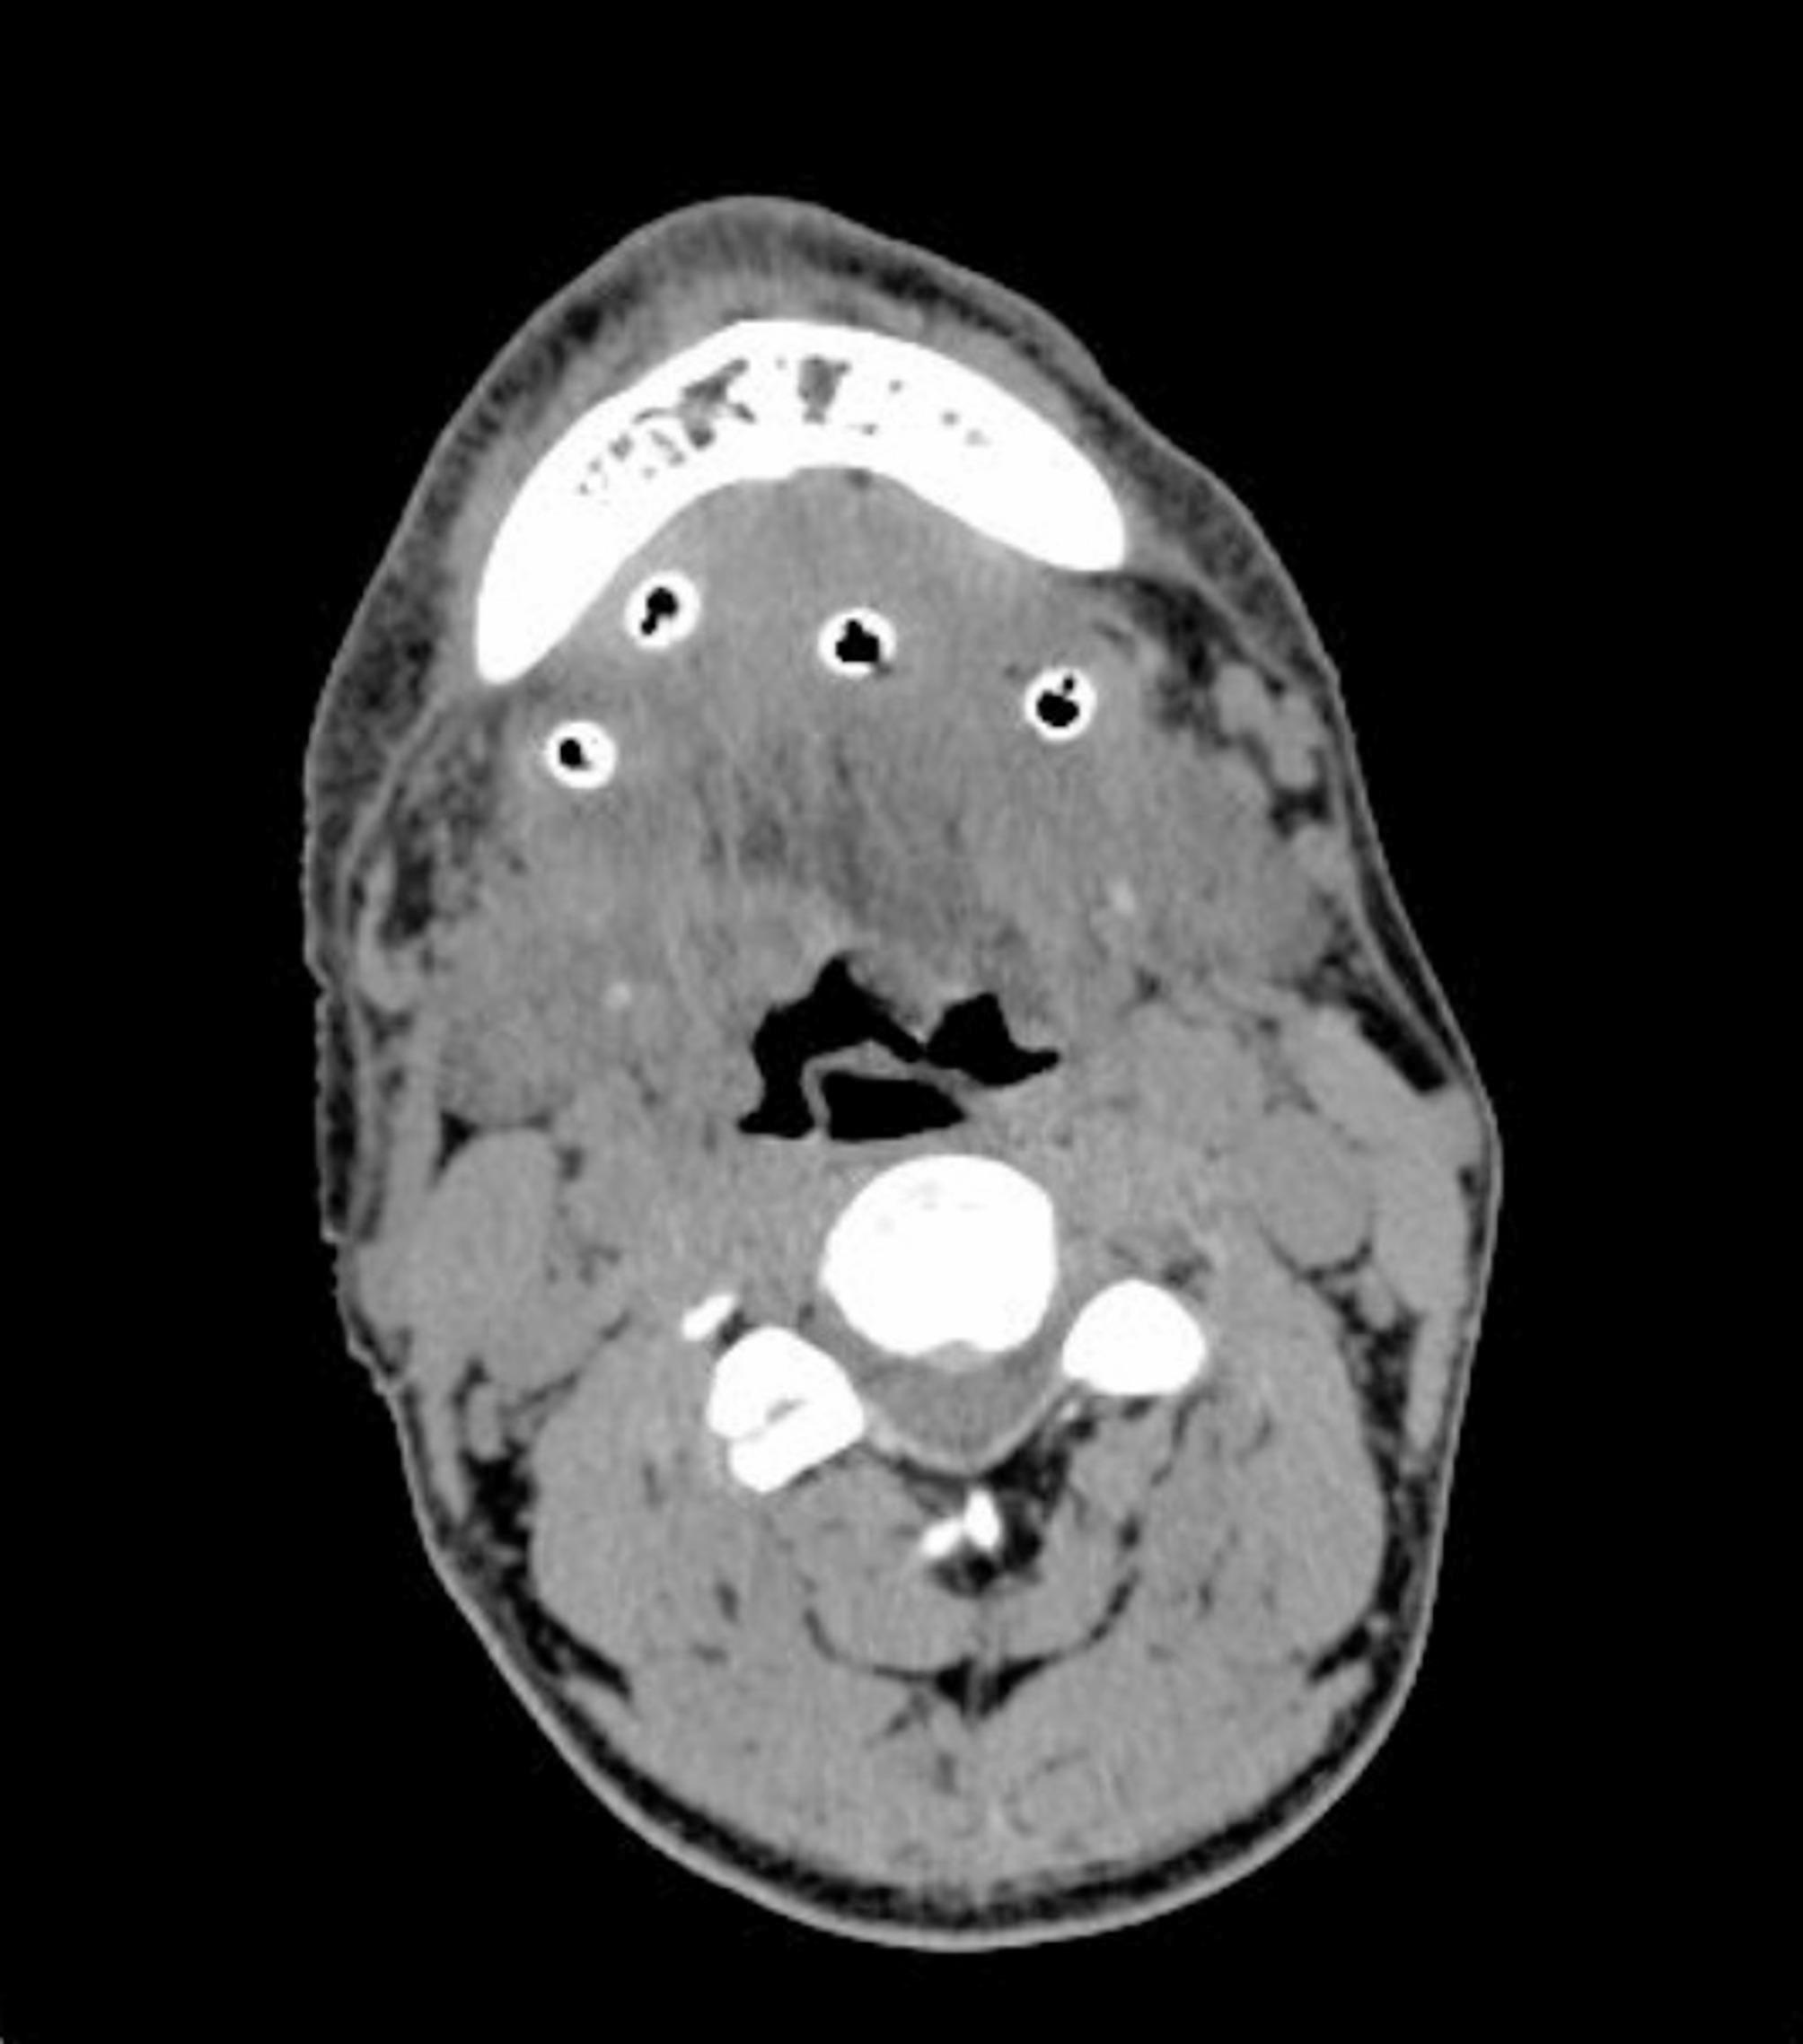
A postoperative axial CT image showed proper NPWT device placement
